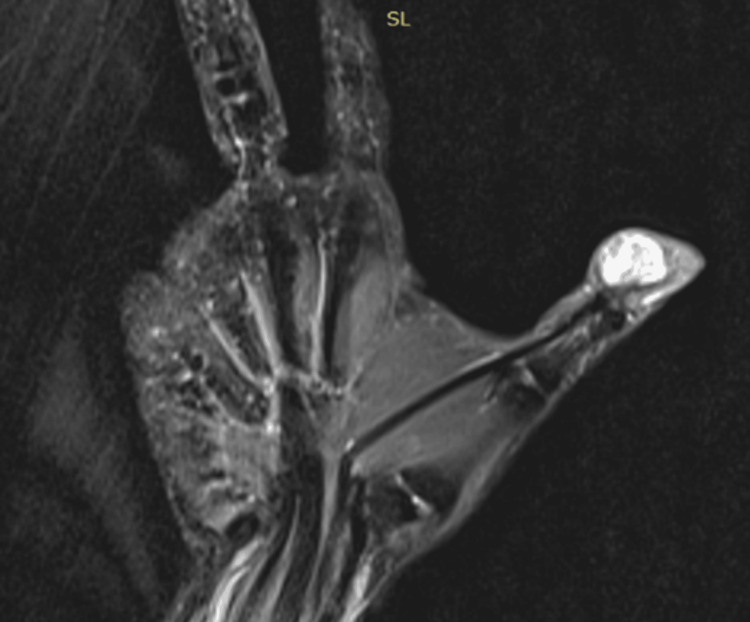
T2-weighted MRI depicts the soft-tissue mass on the palmar surface of the thumb MRI: magnetic resonance imaging
